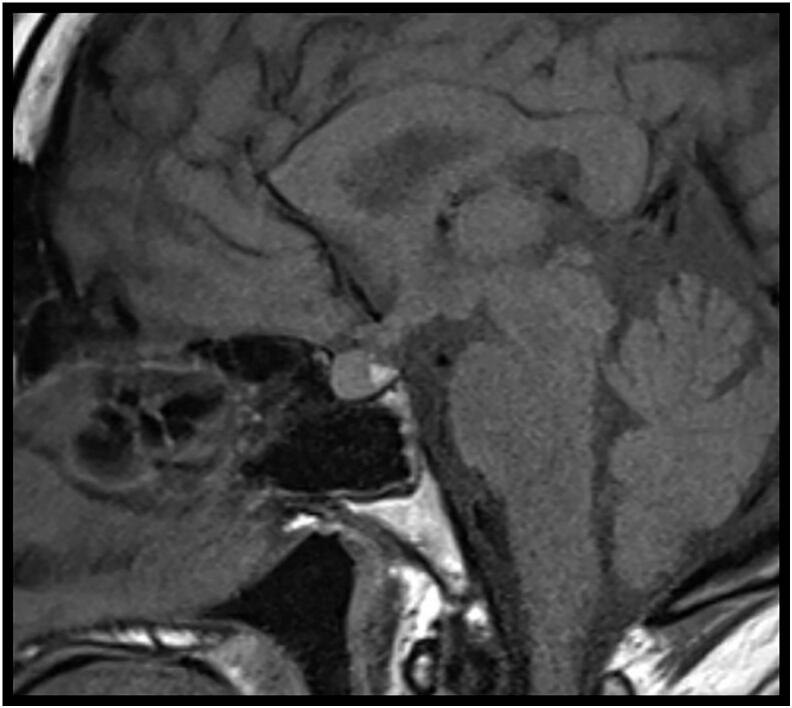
MRI sellar lesion showing pituitary mass with heterogenous signal at T1 sequence with post-contrast enhancement.
